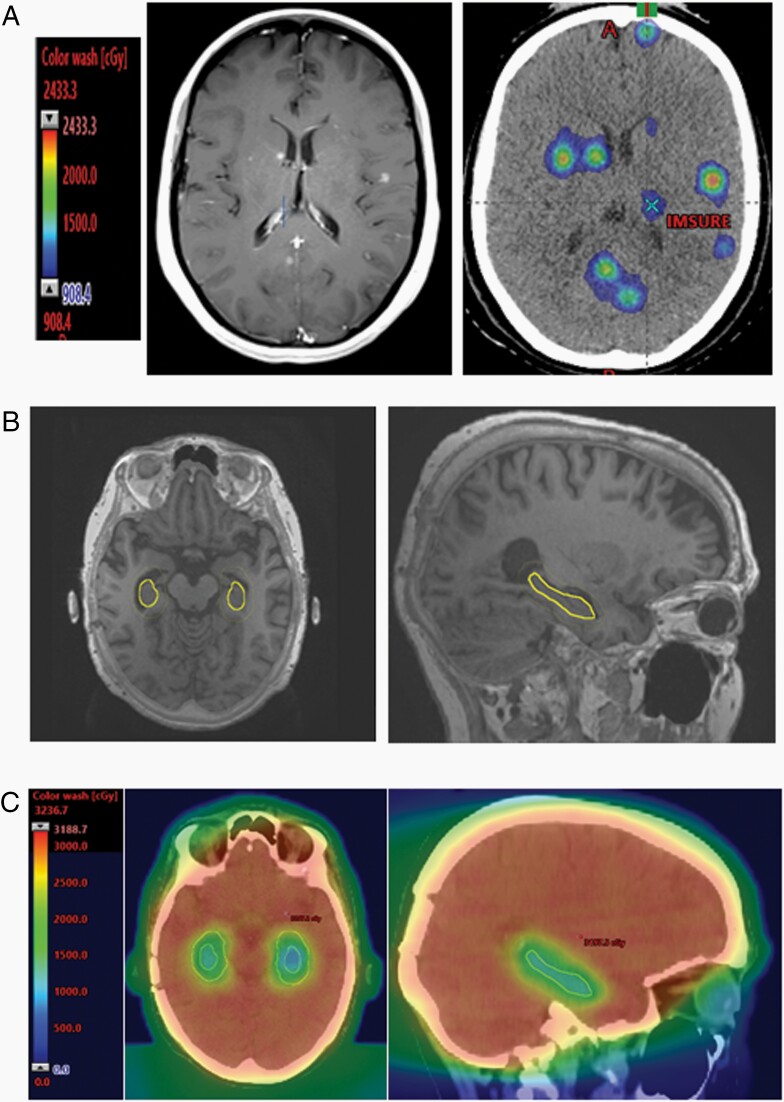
(A) Stereotactic radiosurgery (SRS) radiation treatment plan for multiple metastases shown on T1 contrasted MRI on left with single fraction doses in color wash dose distributions on right. (B) Hippocampal avoidant whole-brain radiotherapy (HA-WBRT): The hippocampal avoidance region (yellow) is generated by expanding the hippocampal contour (thin yellow) by 5 mm. (C) HA-WBRT (30 Gy in 10 fractions) color wash dose distributions are shown on representative axial and sagittal images.
